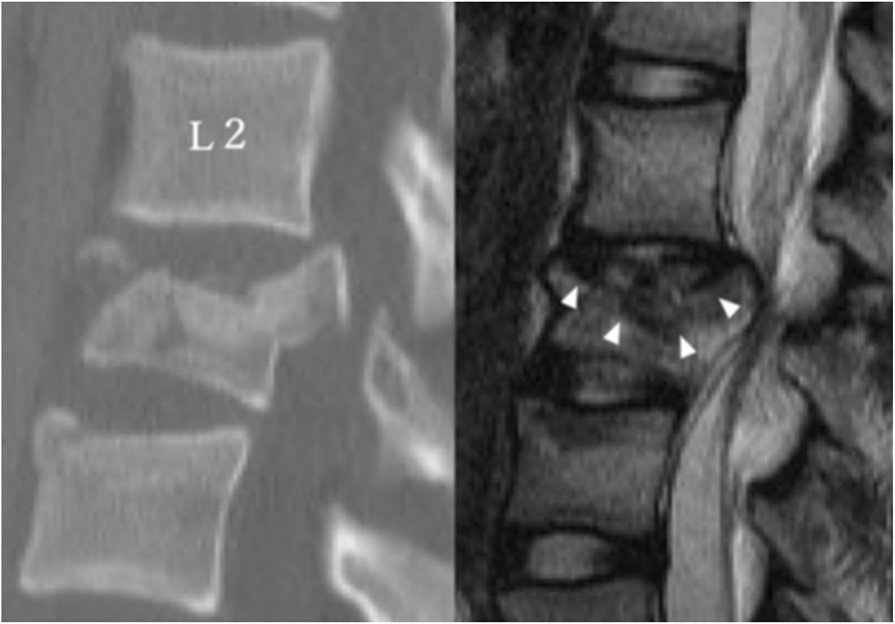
Classification of traumatic intervertebral disc lesion (TIDL). A case of AO type A3 fracture at L3. CT shows a fracture of the cranial endplate and MRI shows infraction of the disc into the vertebral body (white triangles) which means a TIDL grade 3. The caudal disc showed a TIDL grade 2
